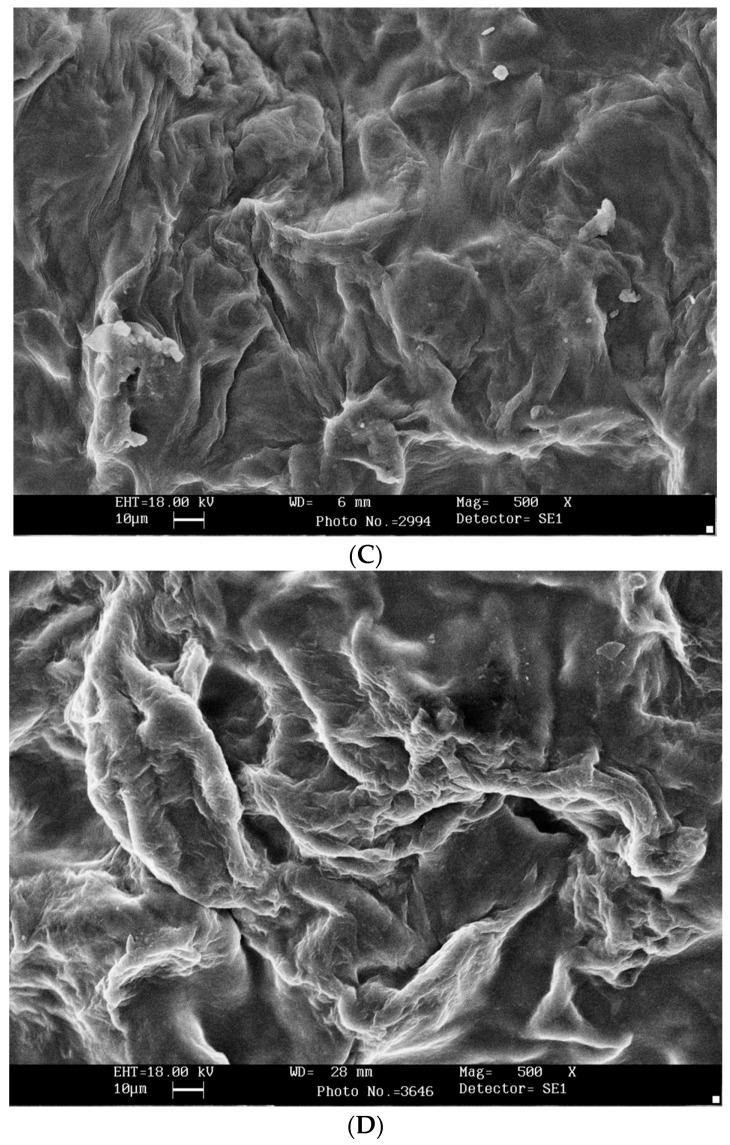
SEM images of surface of convectively dried banana slices pretreated with (A) ultrasonic power of 0 W–sample mass to coating solution mass ratio of 1:2, (B) ultrasonic power of 1000 W–sample mass to coating solution mass ratio of 1:2, (C) ultrasonic power of 0 W–sample mass to coating solution mass ratio of 1:4, and (D) ultrasonic power of 1000 W–sample mass to coating solution mass ratio of 1:4.
